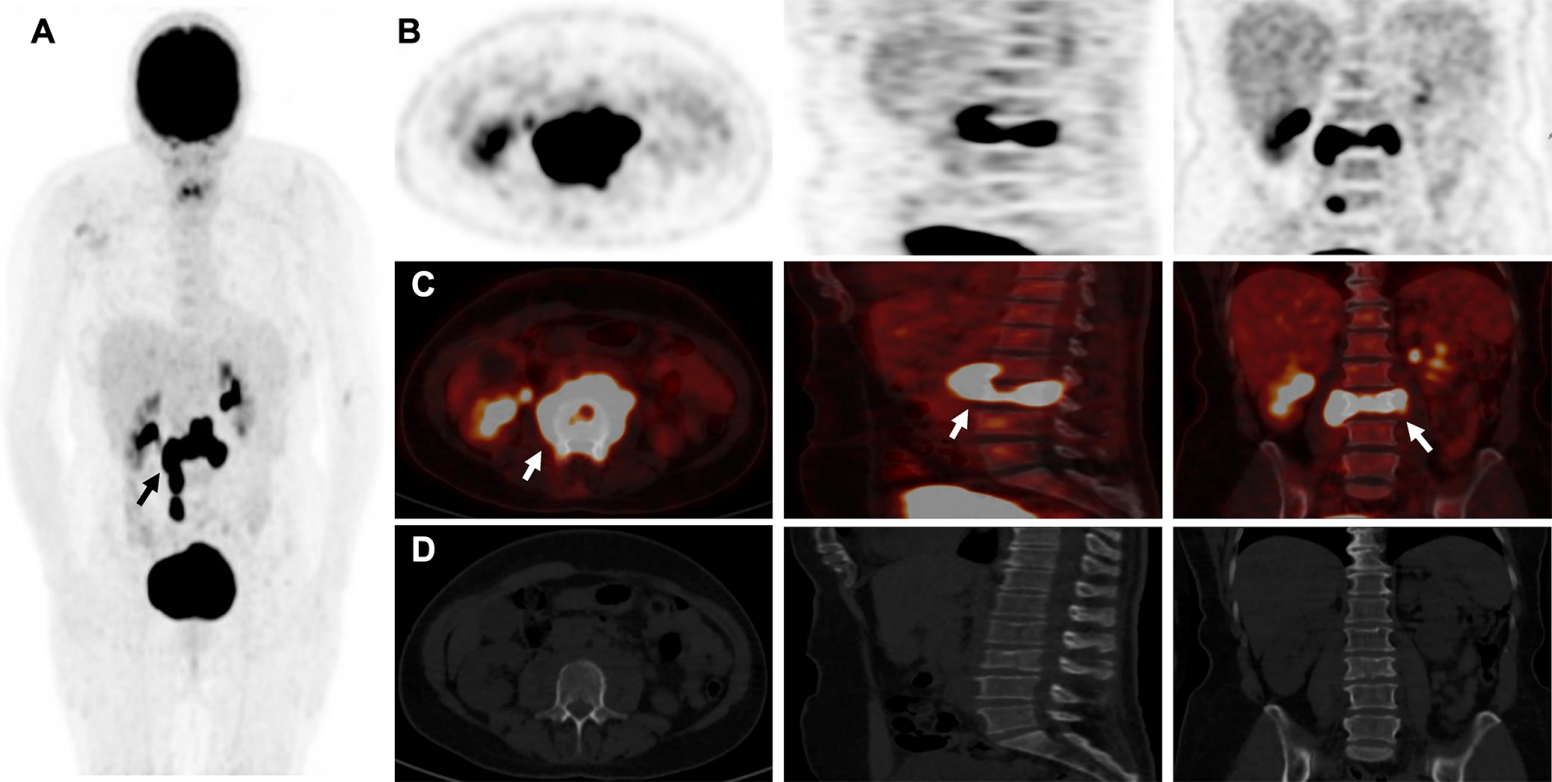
^18^F-FDG-PETCT showing higher uptake and extensive invasion at the L3 vertebral level and questionable invasion at the L3-L4 intervertebral disc level (white arrow), with an “inflammatory cast” appearance (black arrow) and more aggressive metabolic extension anteriorly and posteriorly in maximum intensity projection (MIP) (A,B) and merged images (C) than CT (D) in addition, no other lesions were found in the rest of the body explored.
